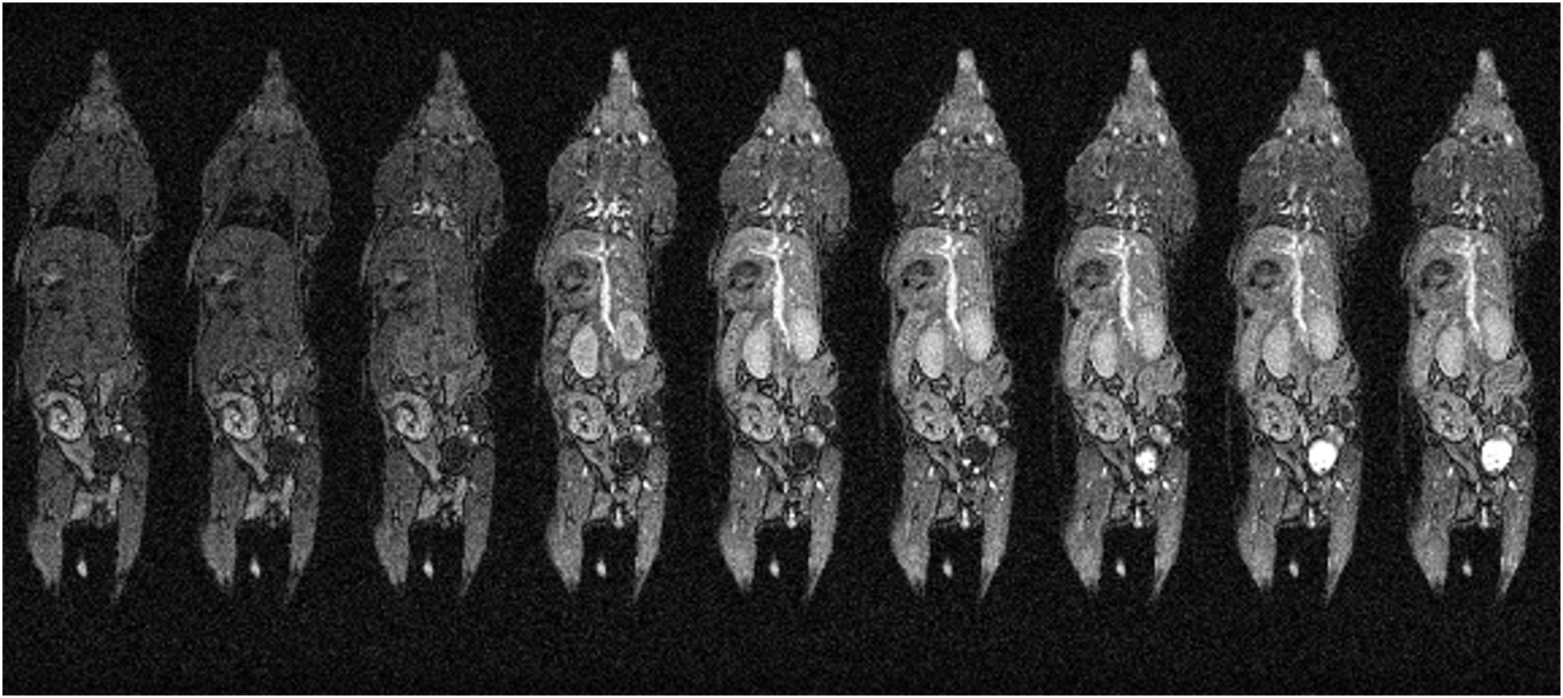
Coronal image slice from volumes 9, 10, 11, 12, 15, 20, 30, 40 and 50 taken from a 3D DCE-MRI time course. Gd-contrast agent was infused via a tail vein cannula over 15 s starting at the beginning of volume 11. 3D data volumes were acquired every 12–15 s.
